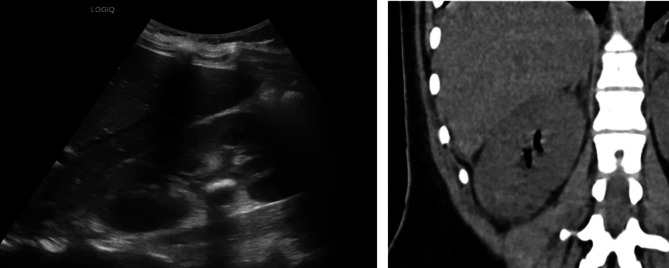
Left:* Abdominal ultrasound demonstrating right kidney calculus, with minimal hydronephrosis. *Right*: Non-contrast computed tomography showing air in the collecting system of the right kidney, consistent with class 1 emphysematous pyelonephritis, defined as gas confined to the collecting system only [1]
